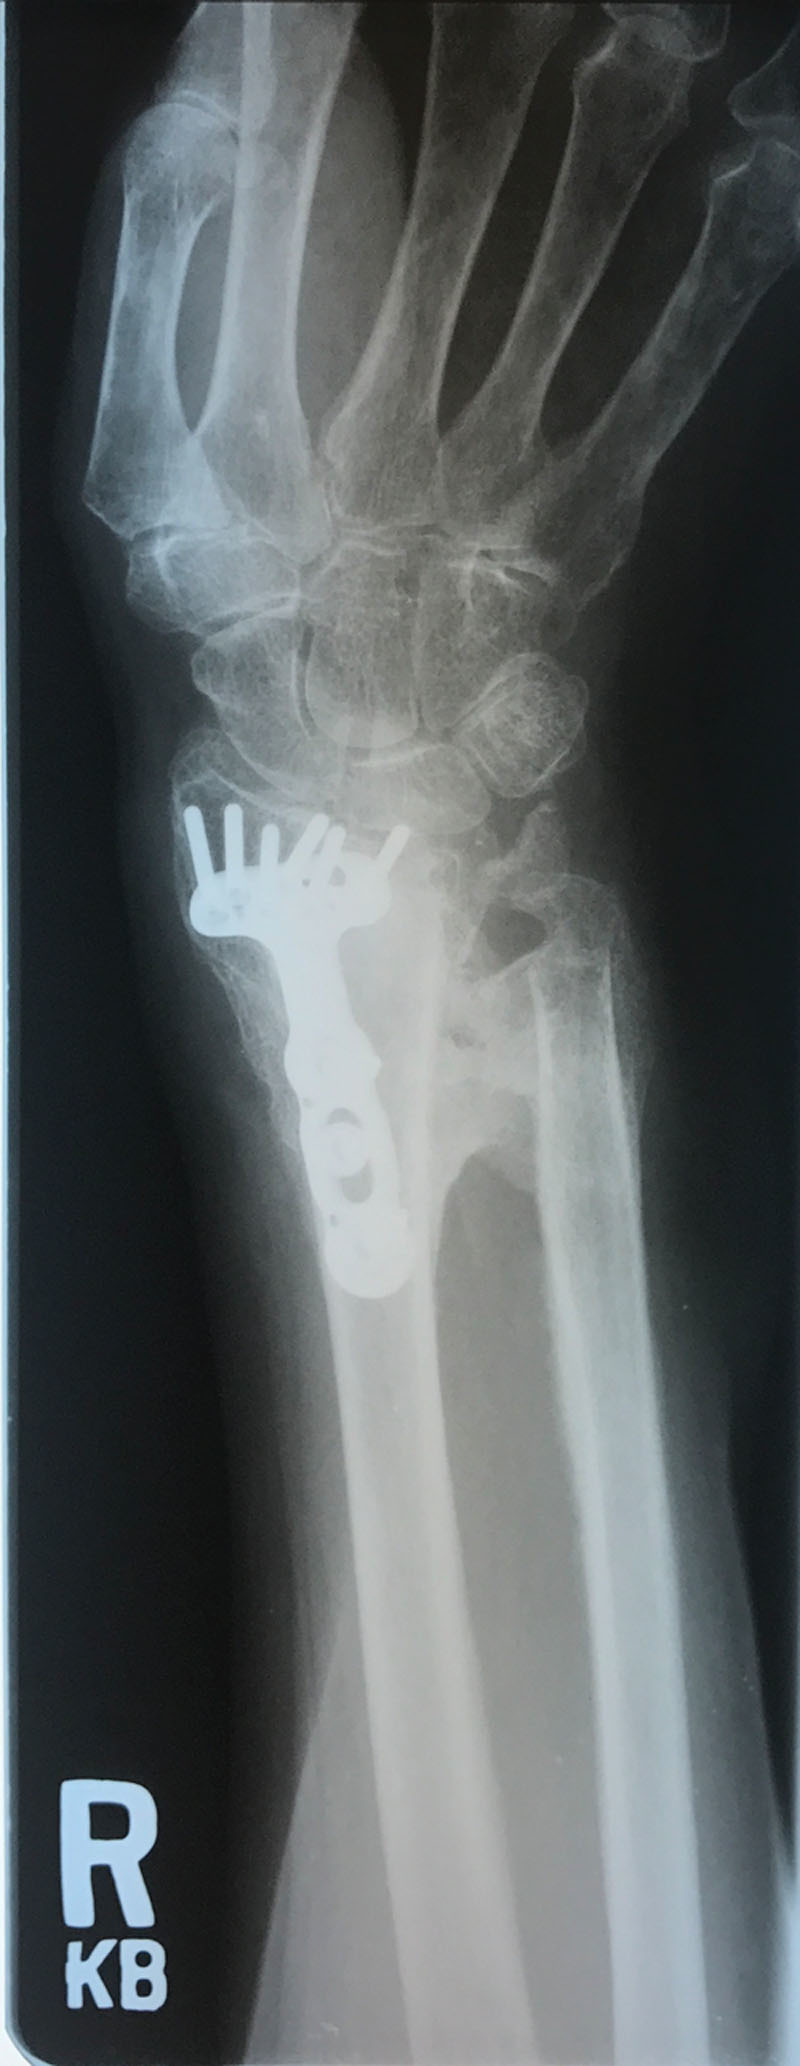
Patient 2 preoperative roentgenogram showing heterotopic ossification of the right distal radius and ulna.
